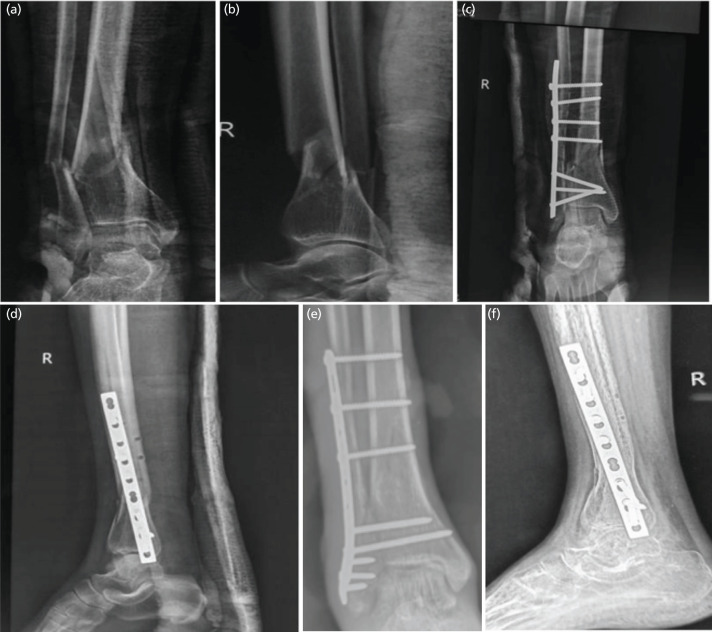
Radiographs of 30-year-old patient with distal tibial fracture managed with fibula pro tibia, (a, b) pre-operative, (c, d) immediate post-op, and (e, f) after one year.
